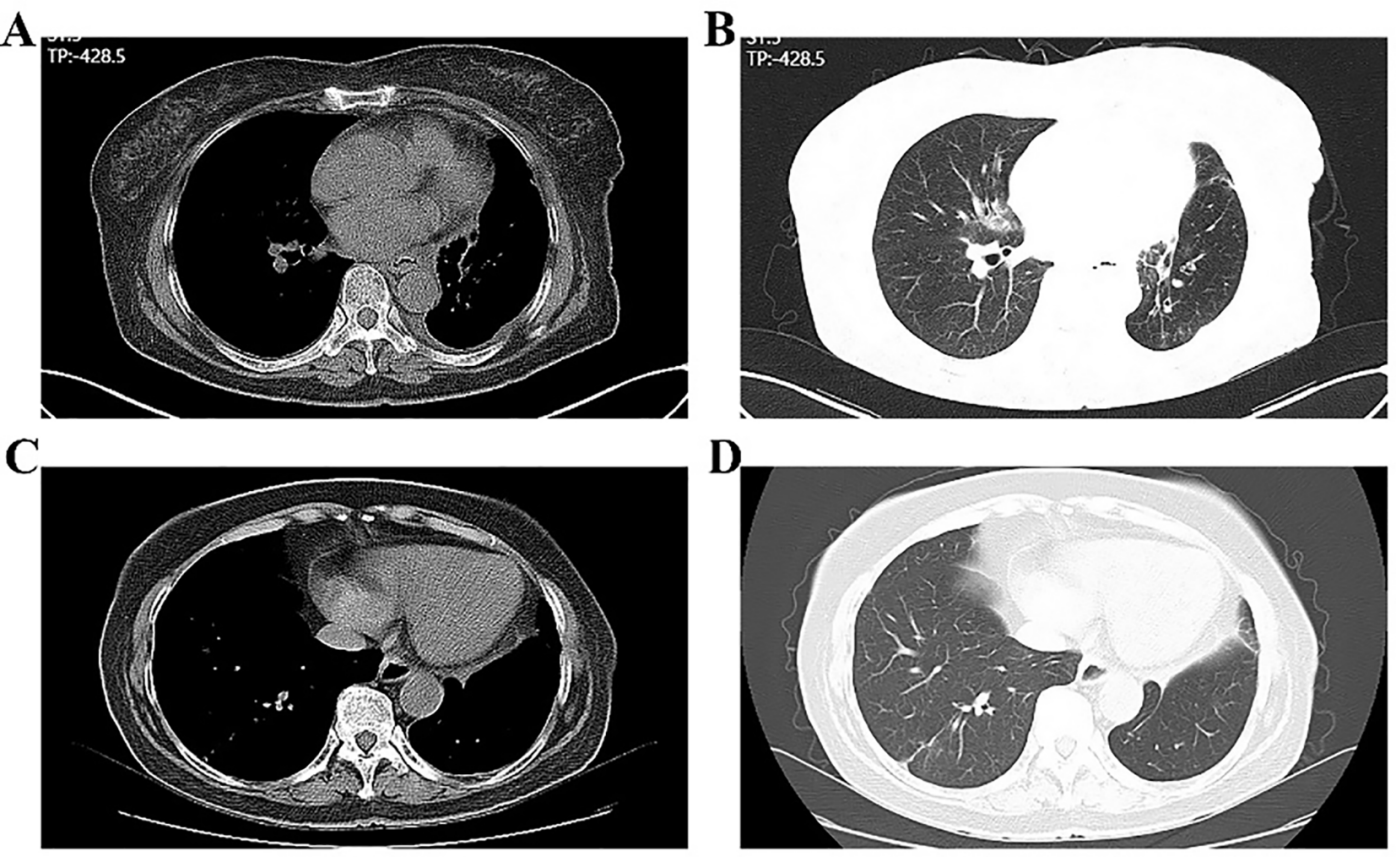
Postoperative plain chest CT of the patient. (A, B) Before discharge; (C, D) One month after discharge.
